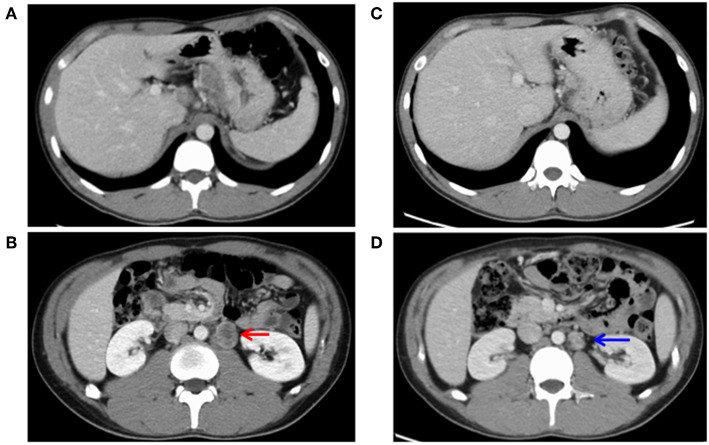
Images of computed tomography before (A,B) and after (C,D) chemotherapy. (A) Cardiac wall thickness and connected lymph node swelling was observed. (B) Paraaortic lymph node metastasis (#16) showed distant metastasis of 30 mm in size (indicated by a red arrow). (C) The lymph node of the gastric cardia had shrunk. (D) The paraaortic lymph node metastasis (#16) had shurunk to 19 mm in size (a blue arrow), showing a partial response.
